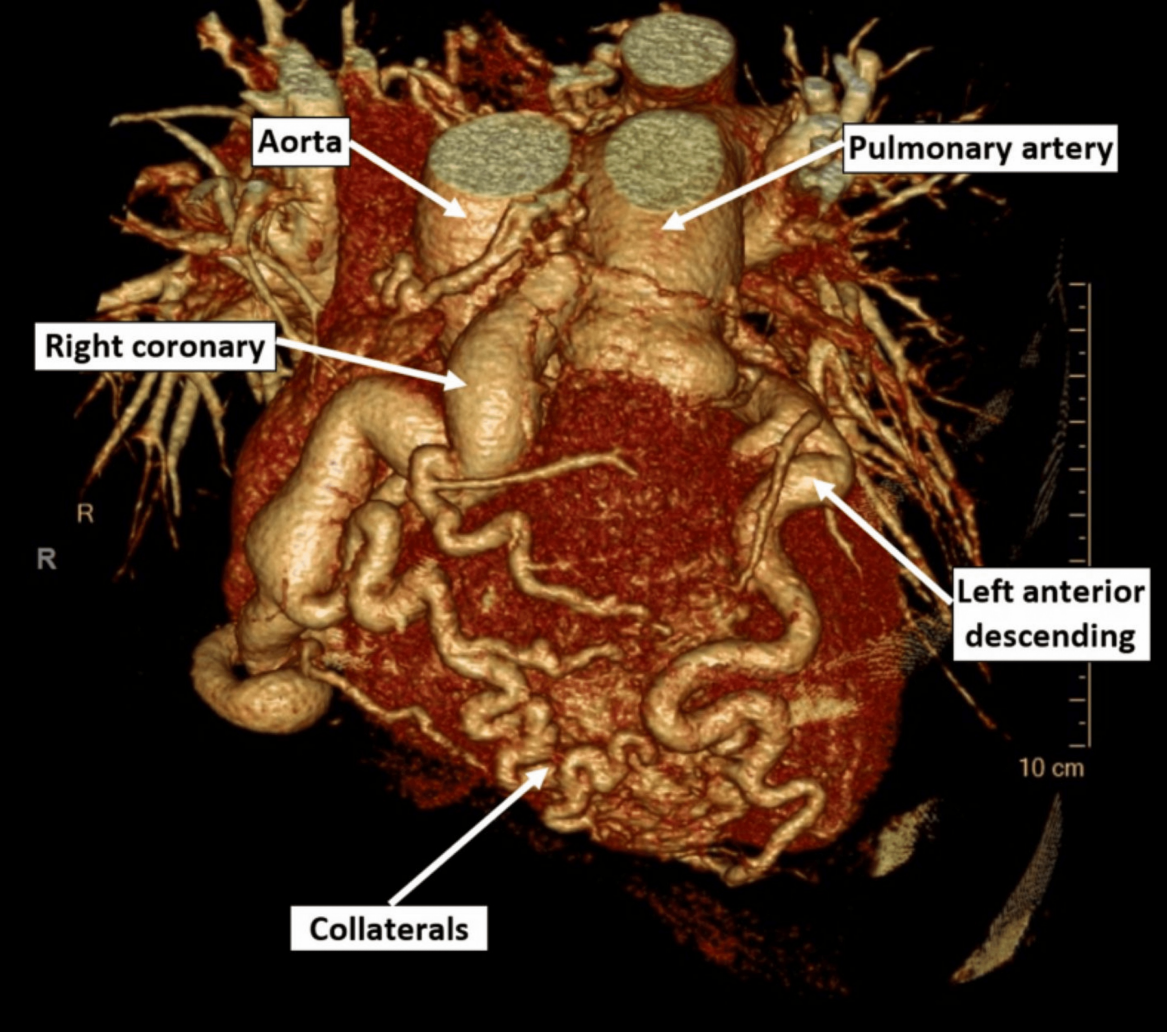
Cardiac CTA of a patient with ALCAPA. Cardiac CTA demonstrates collaterals from the right coronary artery to the left ventricle [29]. Reproduced from Liu et al., CJC Open (2023), distributed under the Creative Commons Attribution (CC BY) license.
